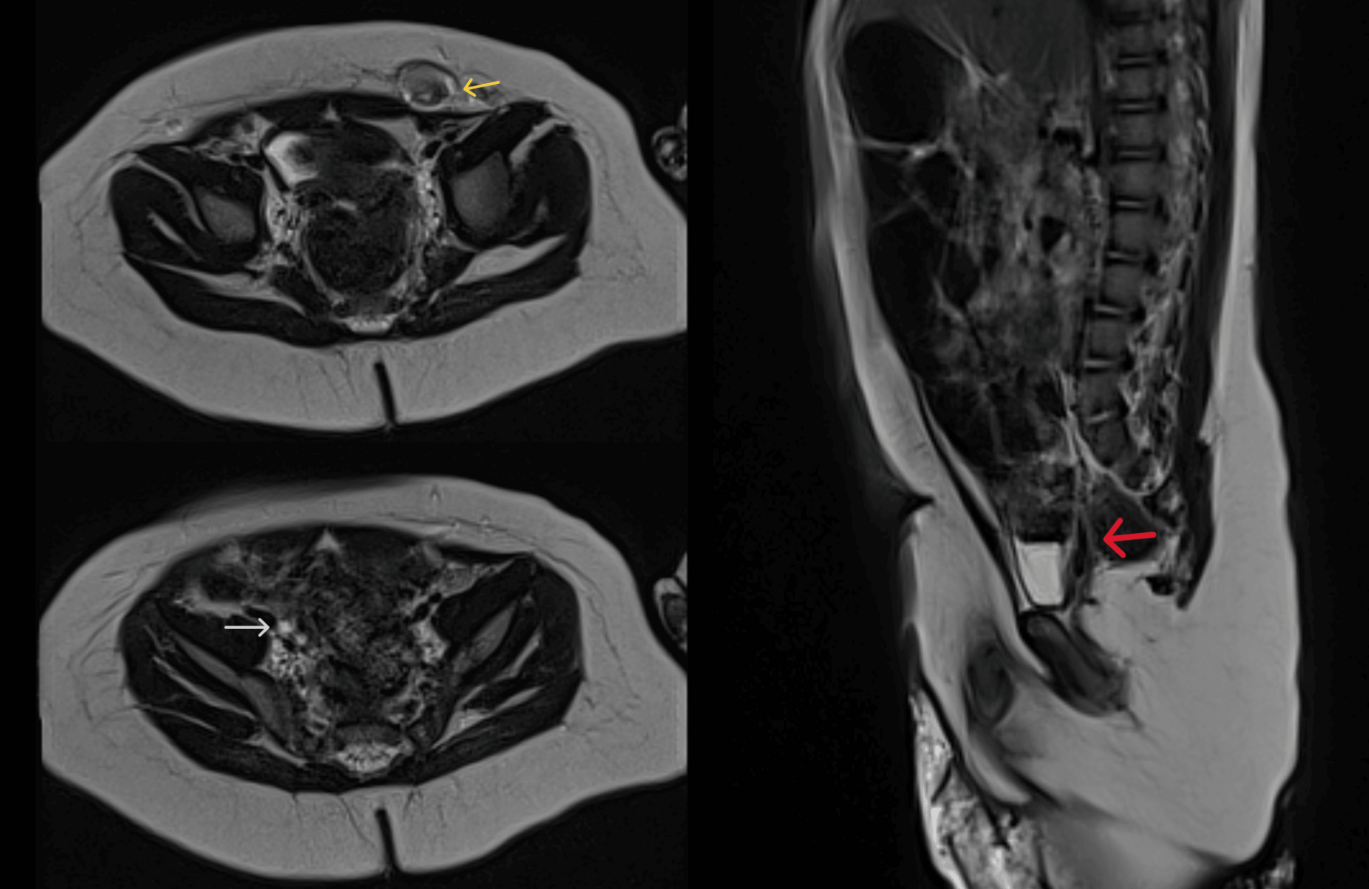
Magnetic resonance imaging highlighting the presence of the uterus (red arrow), left testis (yellow arrow), and right ovarian remnant (white arrow).
